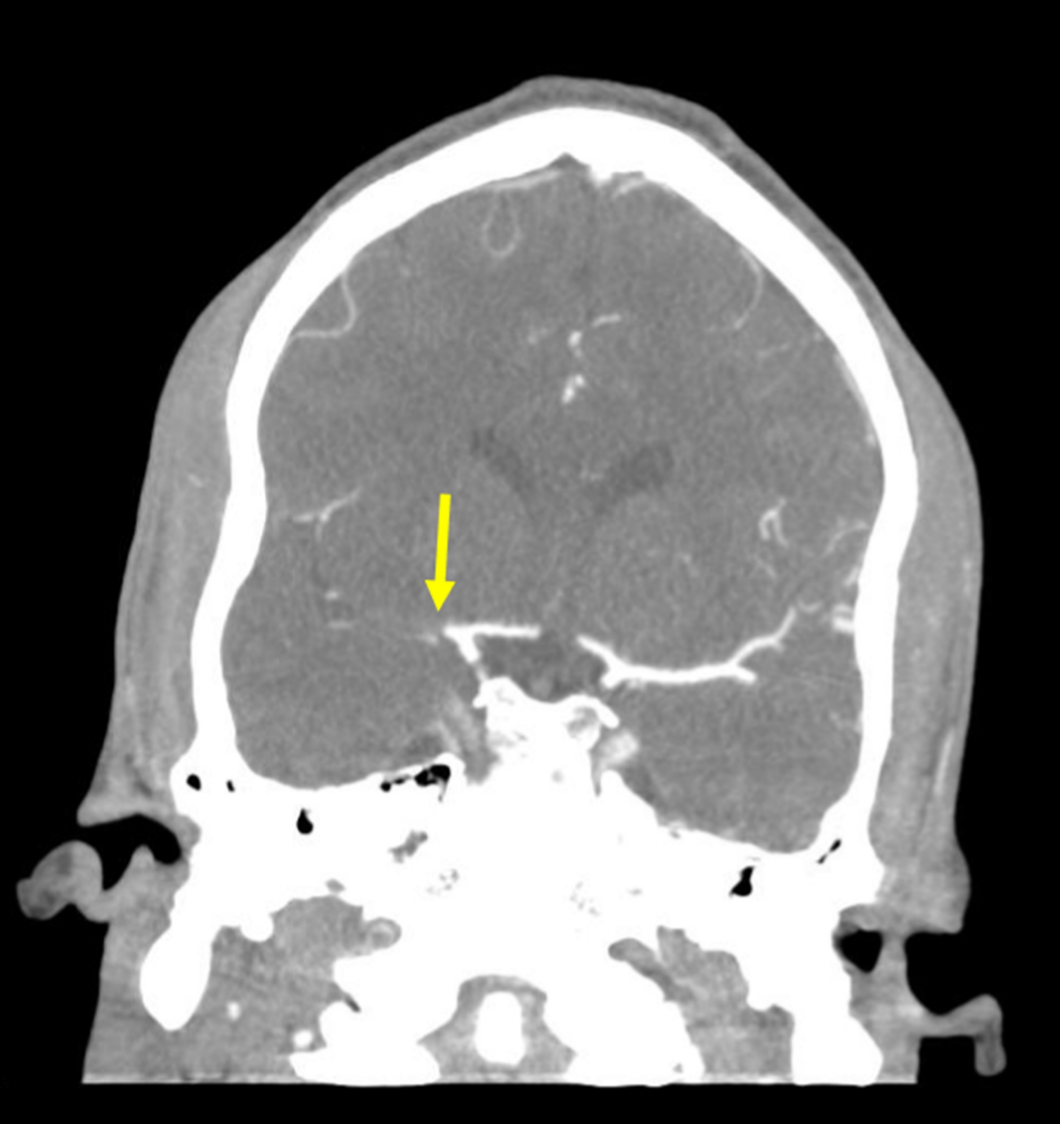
Computed Tomography Angiogram A coronal computed tomography angiogram of the patient's head shows an acute ischemic stroke in the right proximal middle cerebral artery (yellow arrow).
